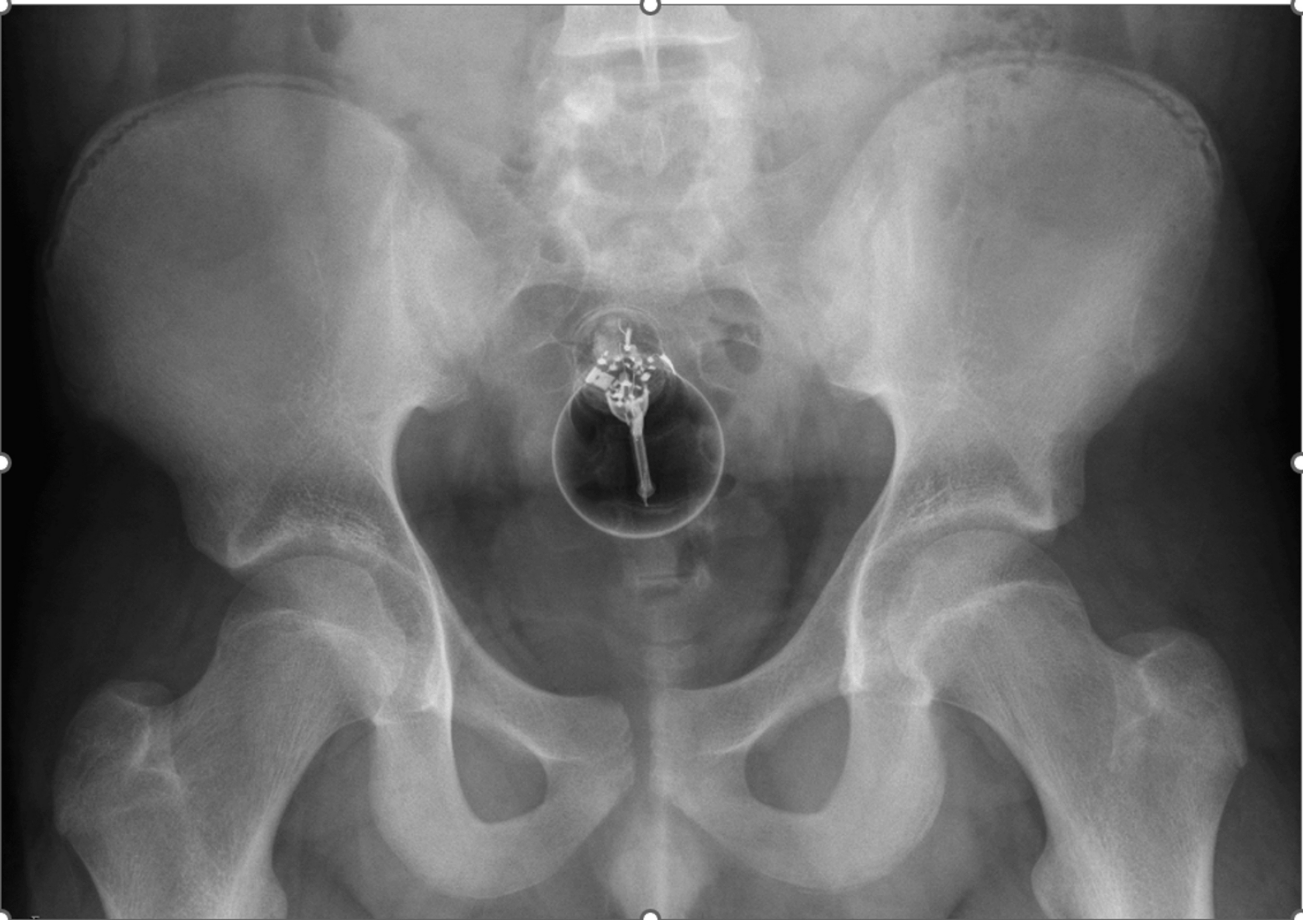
Anteroposterior X-ray of the pelvis showing the foreign body
